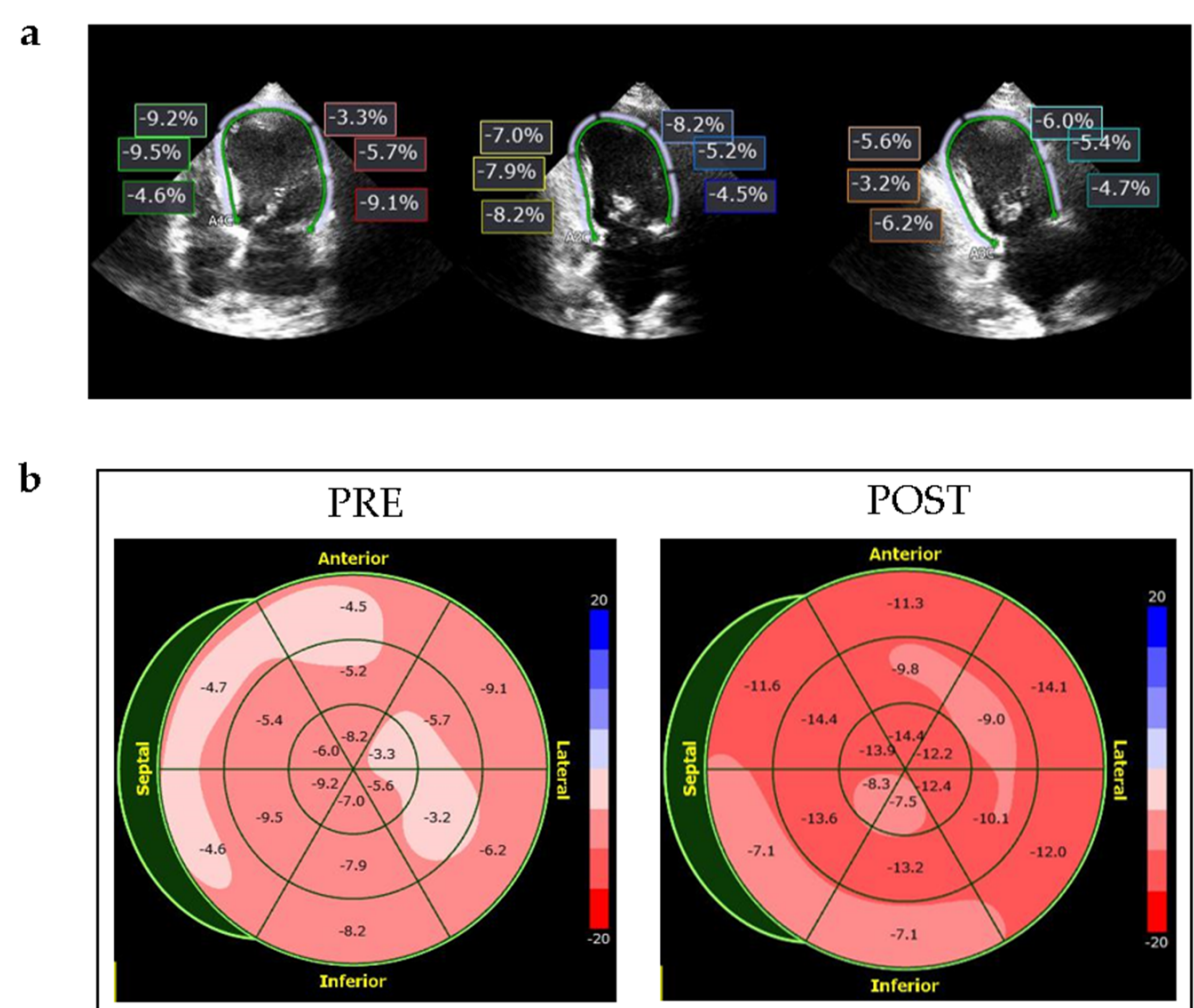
Left ventricular strain in a patient with tachycardic AF and reduced EF. (a) Two-dimensional echocardiographic images showing strain measures of the left ventricle before electrical cardioversion (ECV) in the apical four, three and two chamber view from left to right; (b) 18 segment bull’s eye plots of this patient before (pre) and after (post) ECV. AF: atrial fibrillation; EF: ejection fraction.
